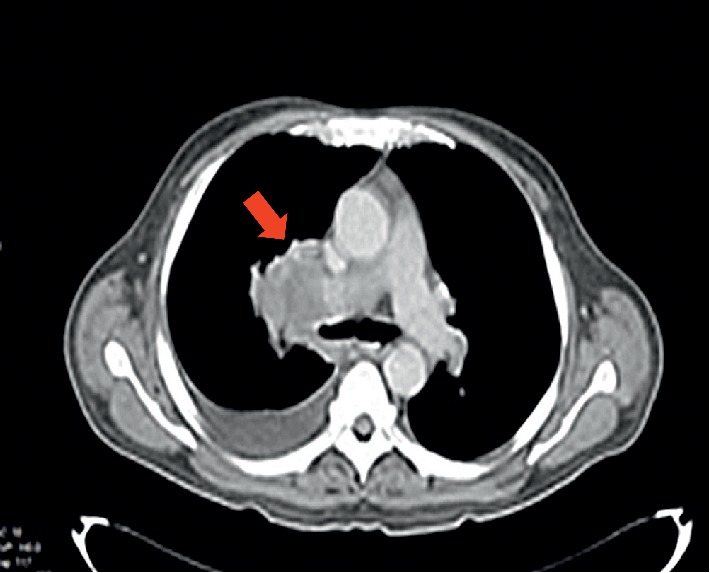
CT thorax showed a right upper intrabronchial neoplastic lesion (red arrow) with right pleural effusion.
